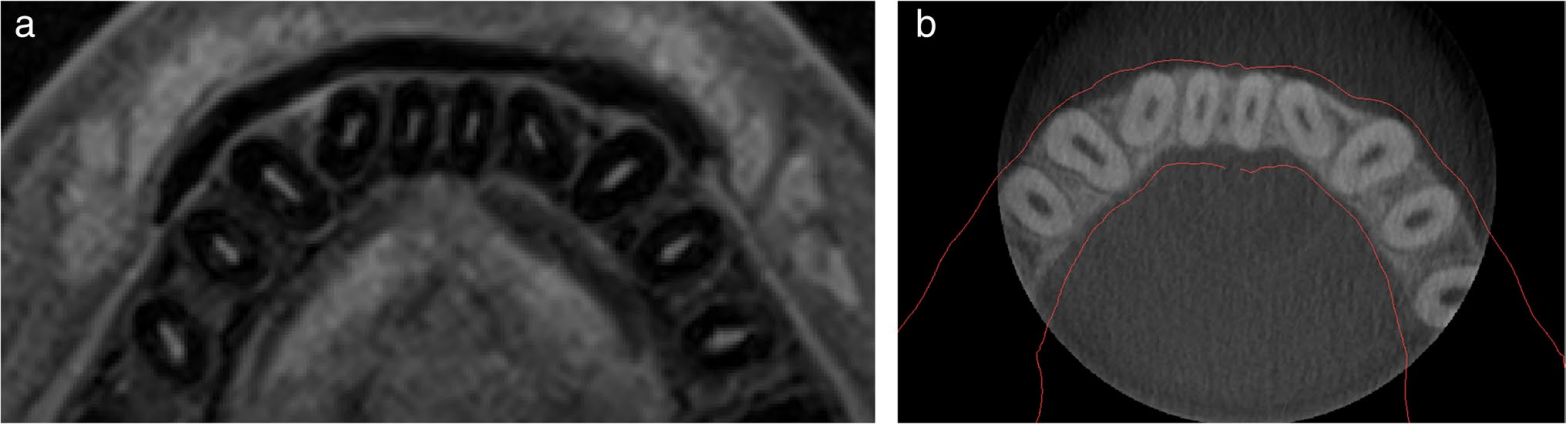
Example of analyzed image datasets: a horizontal plane of MRI; b horizontal plane of superimposed CBCT/intraoral scan of the same patient
